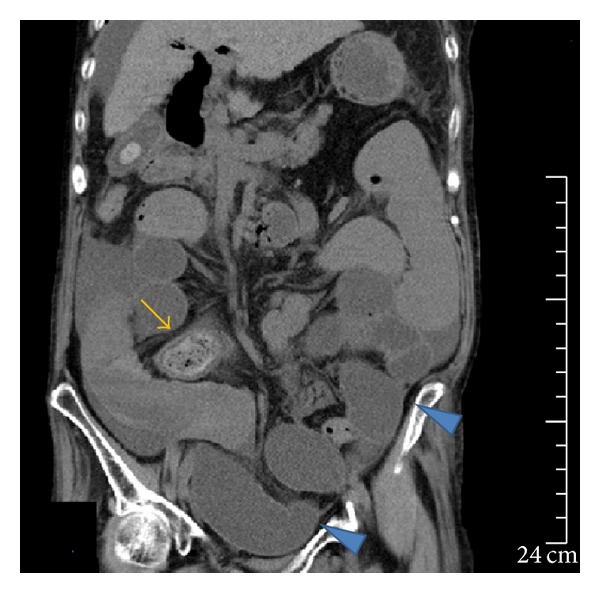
Abdominal CT image showing the stone (arrow) in the ileocecal region on hospital day 59. The stone was successfully passed into the duodenum in the previous ERCP. The image also shows distension of the small intestine proximal to the stone and the accumulation of intraluminal fluid (arrowheads), the latter of which had increased since CT imaging on hospital day 47.
